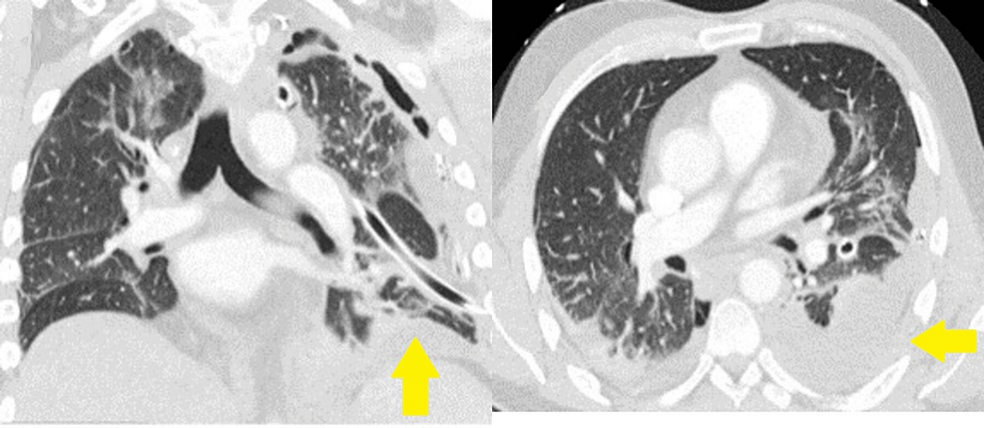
CT scan five days post thoracotomy showing interval improvement in the left-sided hemothorax and retropleural hematoma.
